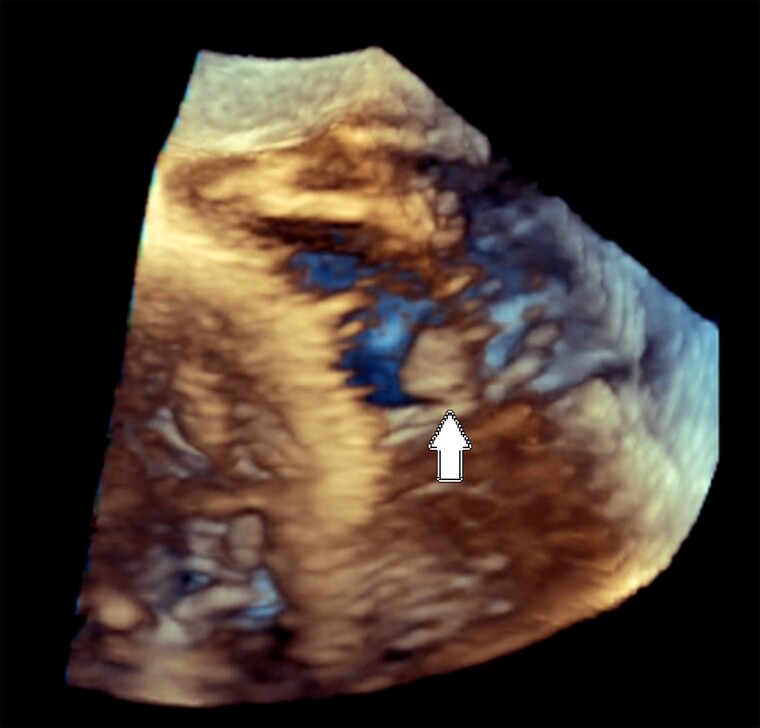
3D elaboration of the tricuspid valve from the transoesophageal transgastric window showing an oval mass attached to the anterior leaflet. The resolution is not enough to catch the true nature of the lesion.
